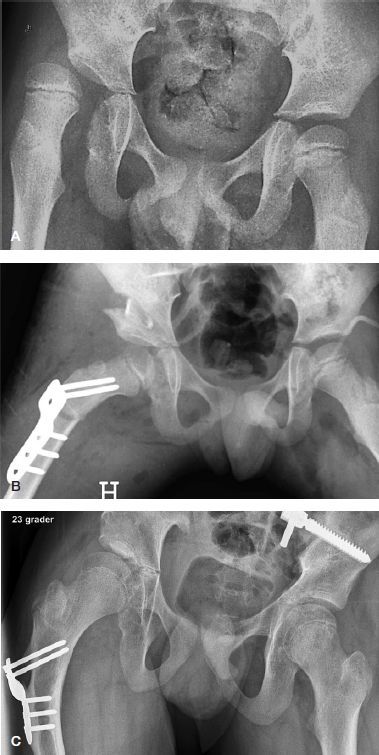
A. Preoperative radiograph of a boy, aged 7.0 years and GMFCS level V, with severe subluxation of his right hip (MP 67%). B. 1 day after femoral osteotomy (varus, derotation and shortening), pelvic osteotomy, and bilateral soft tissue releases, showing good femoral head coverage bilaterally. The cortical bone segment removed from femur has been used as autograft in the open wedge of the pelvic osteotomy. C. 7.4 years postoperatively, at an age of 14.4 years, showing satisfactory femoral head coverage bilaterally, MP 19% (right hip) and 0% (left hip)
